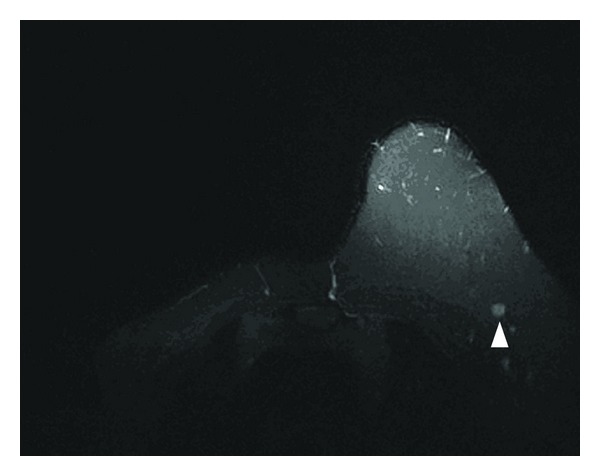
An MRI image demonstrates an axillary reticular mass lesion with T1A hypointense signal intensity changes.
